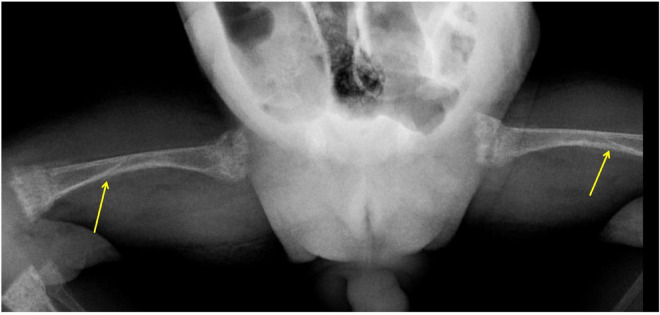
X-Rays of bilateral femurs show shaft fractures (arrows), generalized osteopenia, and metaphyseal changes of rickets (cupping, fraying, and splaying).
